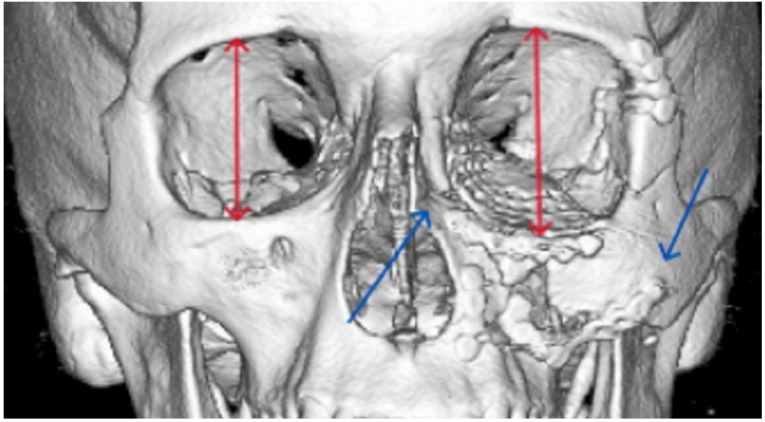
The patient’s left midfacial fractures have been insufficiently reduced. The left malar bone is located inferiorly and rotated laterally, and the medial maxillary and nasal bone fractures are in severe malposition (blue arrows). Although the left orbital floor is reconstructed, the volume of the orbit is increased (red arrows) due to the malposition of the malar bone and the medial maxilla. With the aid of three-dimensional surgical planning and intraoperative CT-scanning, redo surgery could have been avoided in this patient.
